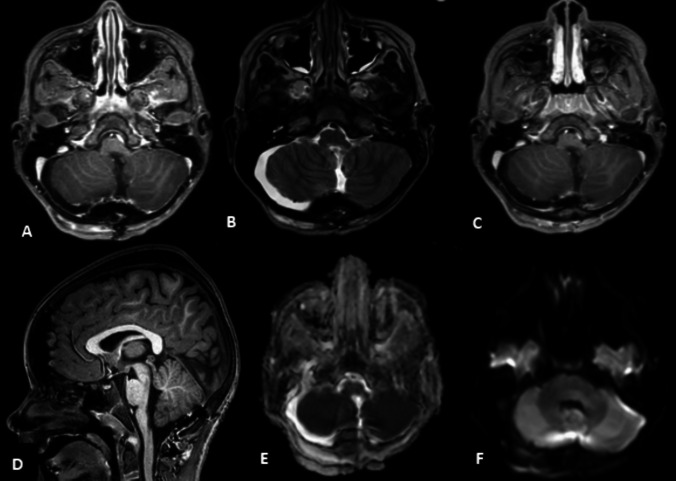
The postoperative MRI findings in the same sequence of Fig. 1 and a total resection with no residual tumor
